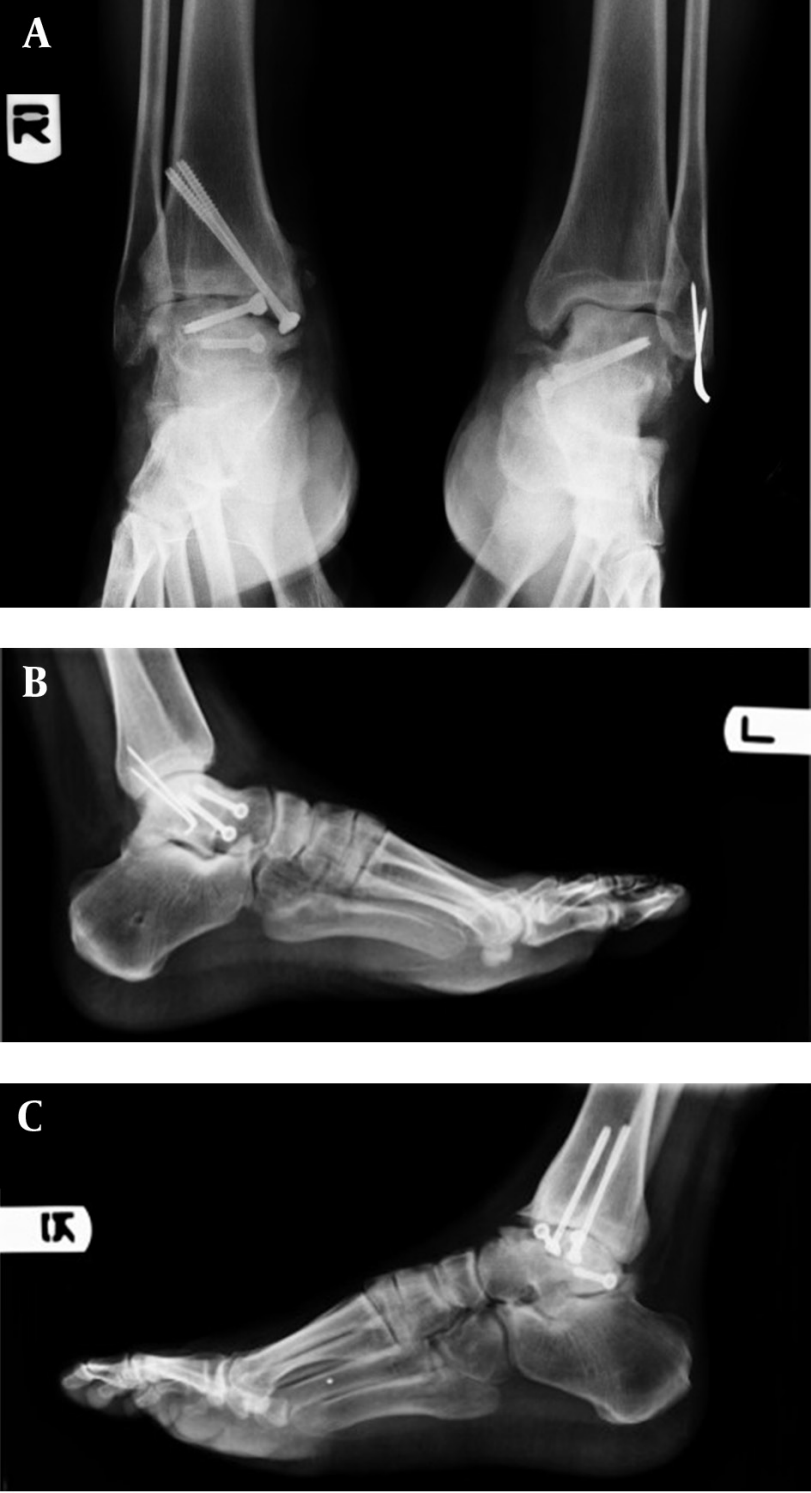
AP and Lateral X-Ray of Right and Left Ankle After Two Years Follow Up
